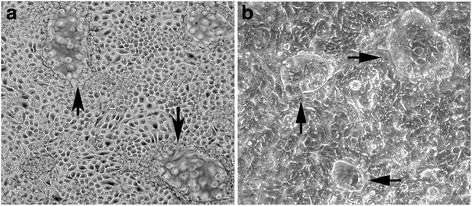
Light level morphology of domes. a. Dome formation in human proximal tubule cells. b. Dome formation in MCF-7 cells expressing the C-terminal domain of MT3 (MT1E-CT). Arrows indicate the presence of domes (both at 100× magnification)
